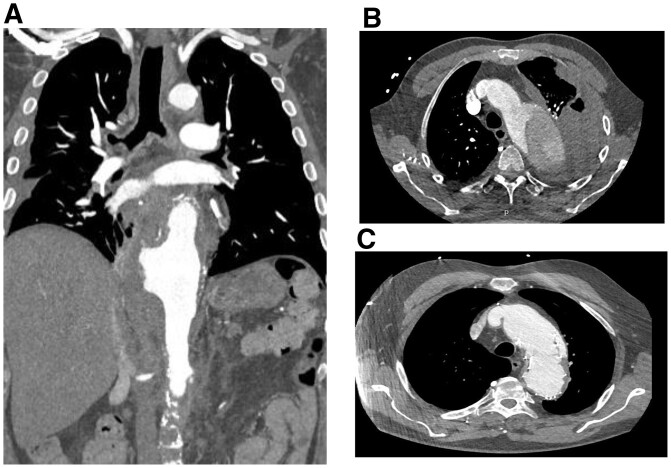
Representative computed tomography angiography scans depicting an aortic rupture (A), a haematothorax caused by an acute aortic rupture (B) and an endoleak type Ia 4 years after emergent thoracic endovascular aortic repair (C).
